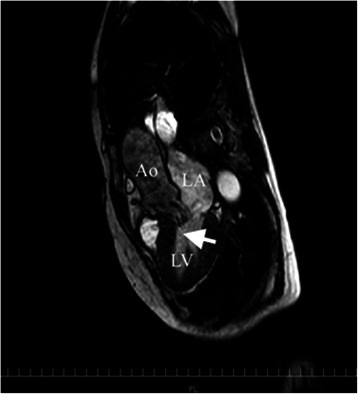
Cardiac MRI obtained 4 days after surgery. It revealed systolic anterior motion (arrow). There were no abnormalities in global shape, size, and systolic function of the left ventricle, except for some protrusion of the basal interventricular septum towards the left ventricular outflow tract. LA = left atrium; LV = left ventricle; Ao = aorta.
